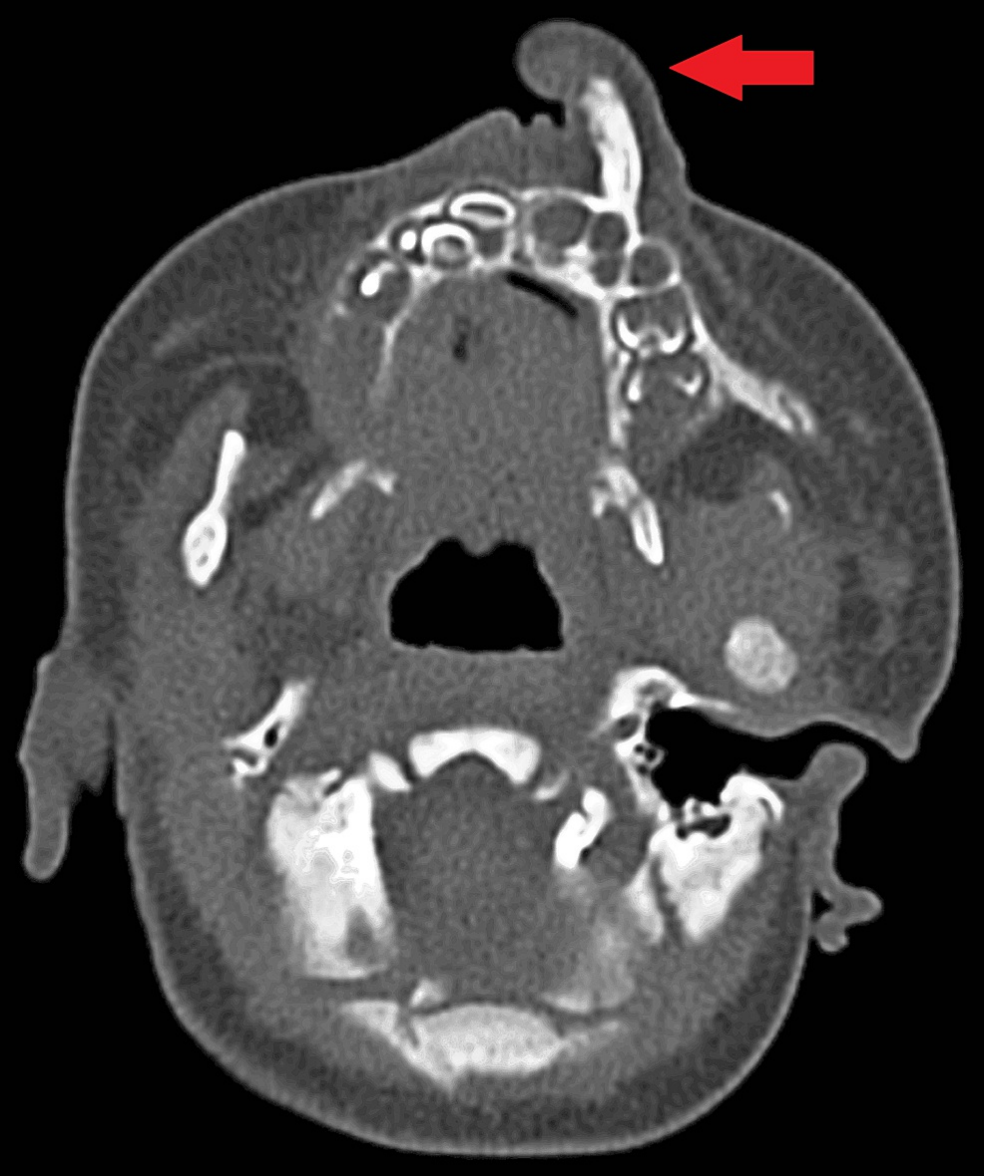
Non-contrast CT brain scan (bone window) The image shows a bony outgrowth protruding from the maxilla with adjacent soft tissue density mass surrounding it. The hard palate appears normal.
